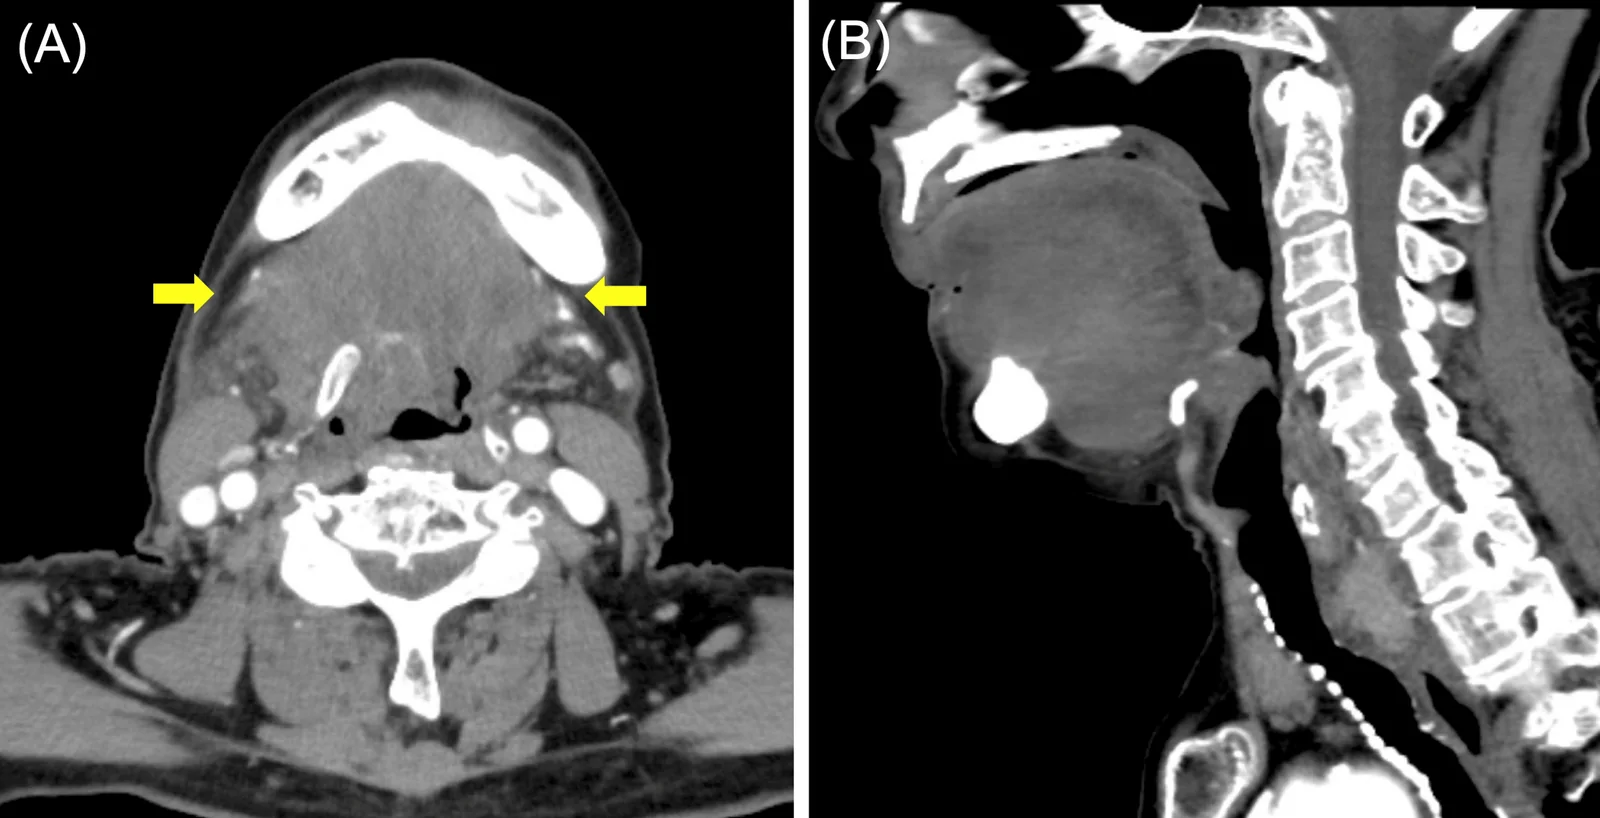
Contrast-enhanced computed tomography (A) Axial contrast-enhanced computed tomography (CT) shows soft tissue swelling extending from the tongue to the floor of the mouth, suggesting a sublingual hematoma (yellow arrow). (B) Coronal contrast-enhanced CT shows the narrowed upper airway.
